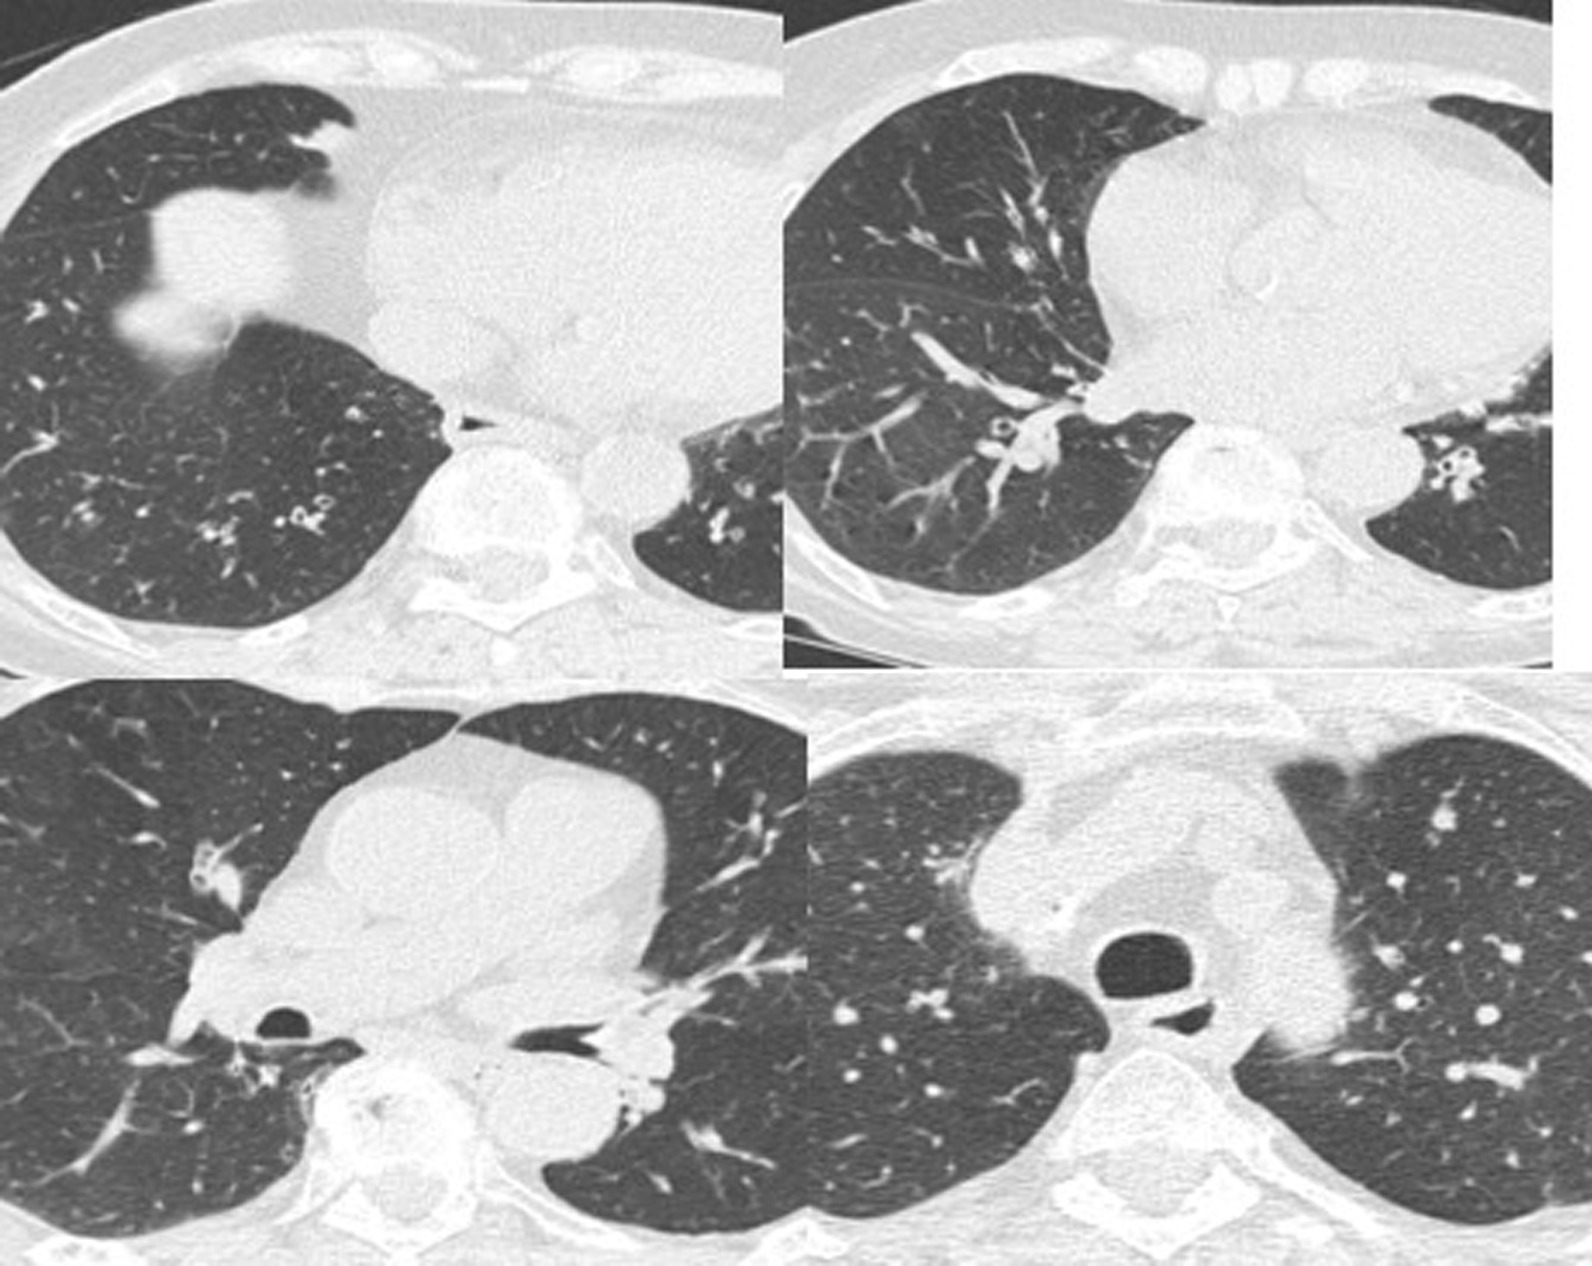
Chest computed tomography scan showed the presence of small areas of air trapping and diffuse bronchiectasis
